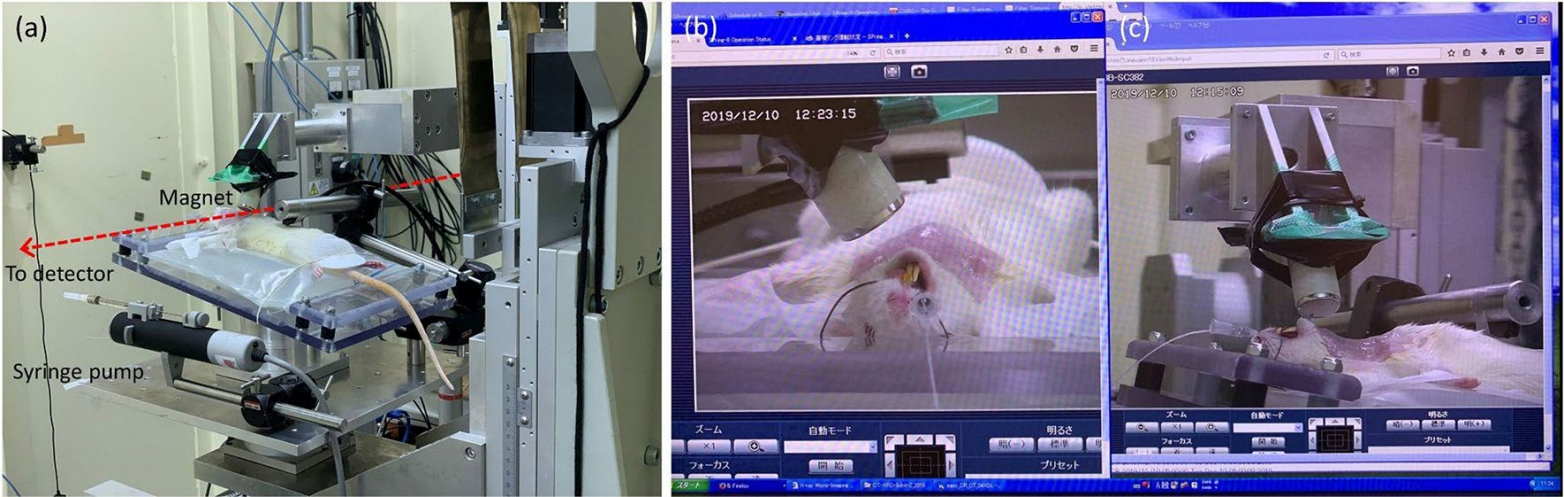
(a) in-vivo imaging setup in the SPring-8 imaging hutch, with the path of the X-ray beam marked with a red dashed line. (b,c) The magnet positioning over the trachea was performed remotely using two orthogonally mounted IP cameras. On the left-hand side of the screen image the wire loop securing the head, and the delivery cannula in position within the ET tube, can be seen.
